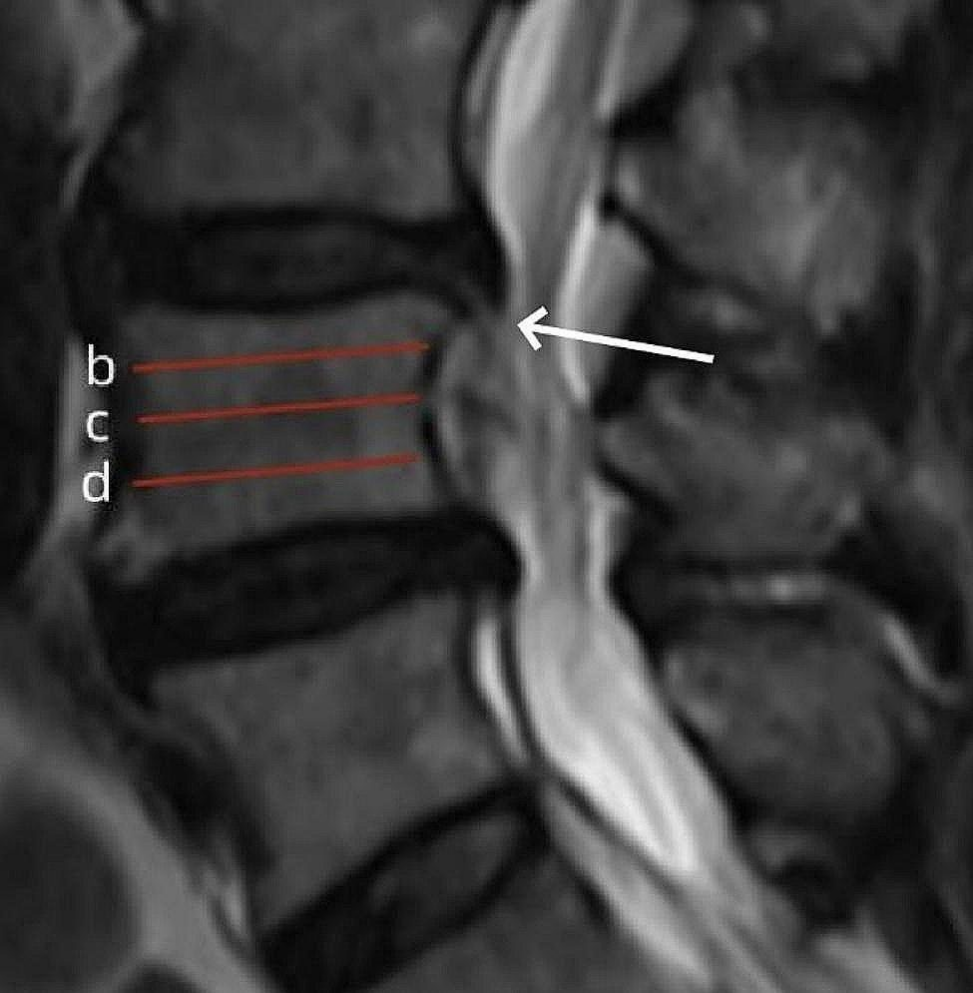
Preoperative evaluation on sagittal view of T2-weighted MRI. Line b, c, and d indicate the boundary for very-highly, highly, and moderately migrated herniation, respectively; the white arrow indicates the ruptured anulus fibrosus
